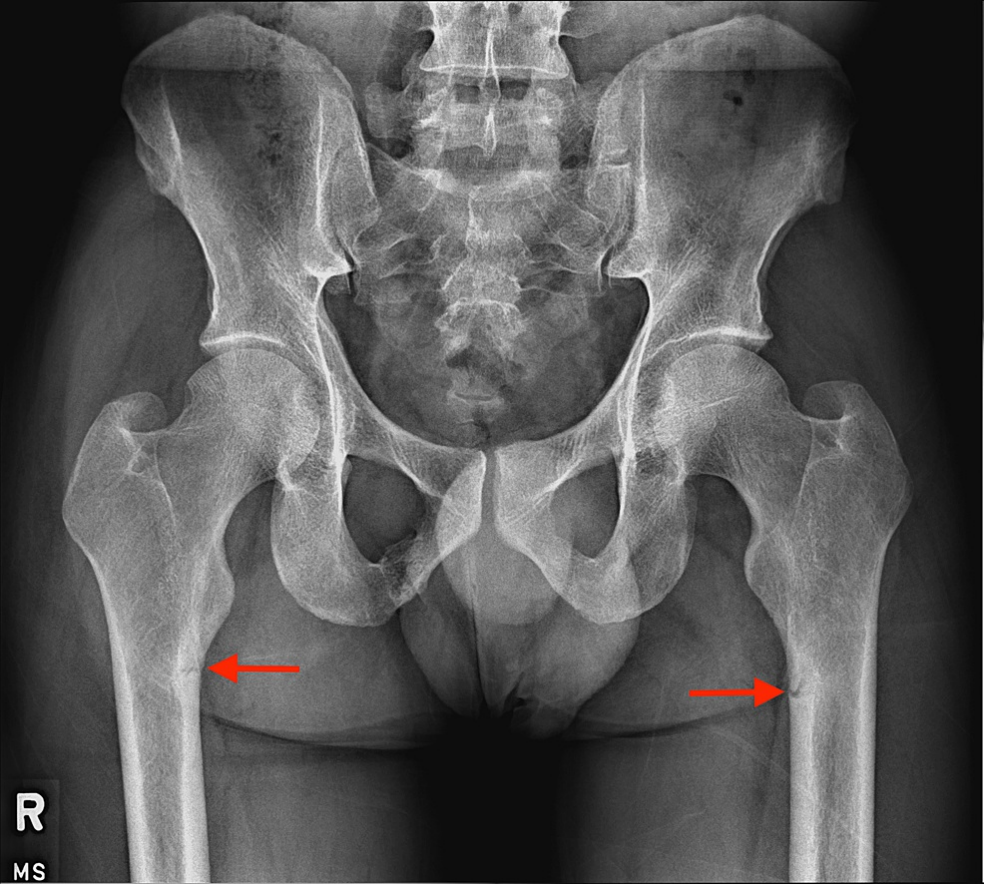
Radiograph of the pelvis and hip showing proximal femoral shaft lucencies indicative of stress fractures
